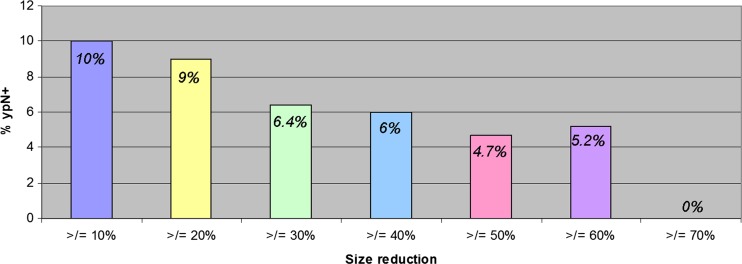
Percentage of positive nodes according to reduction in size between pre- and post-CRT MRI
